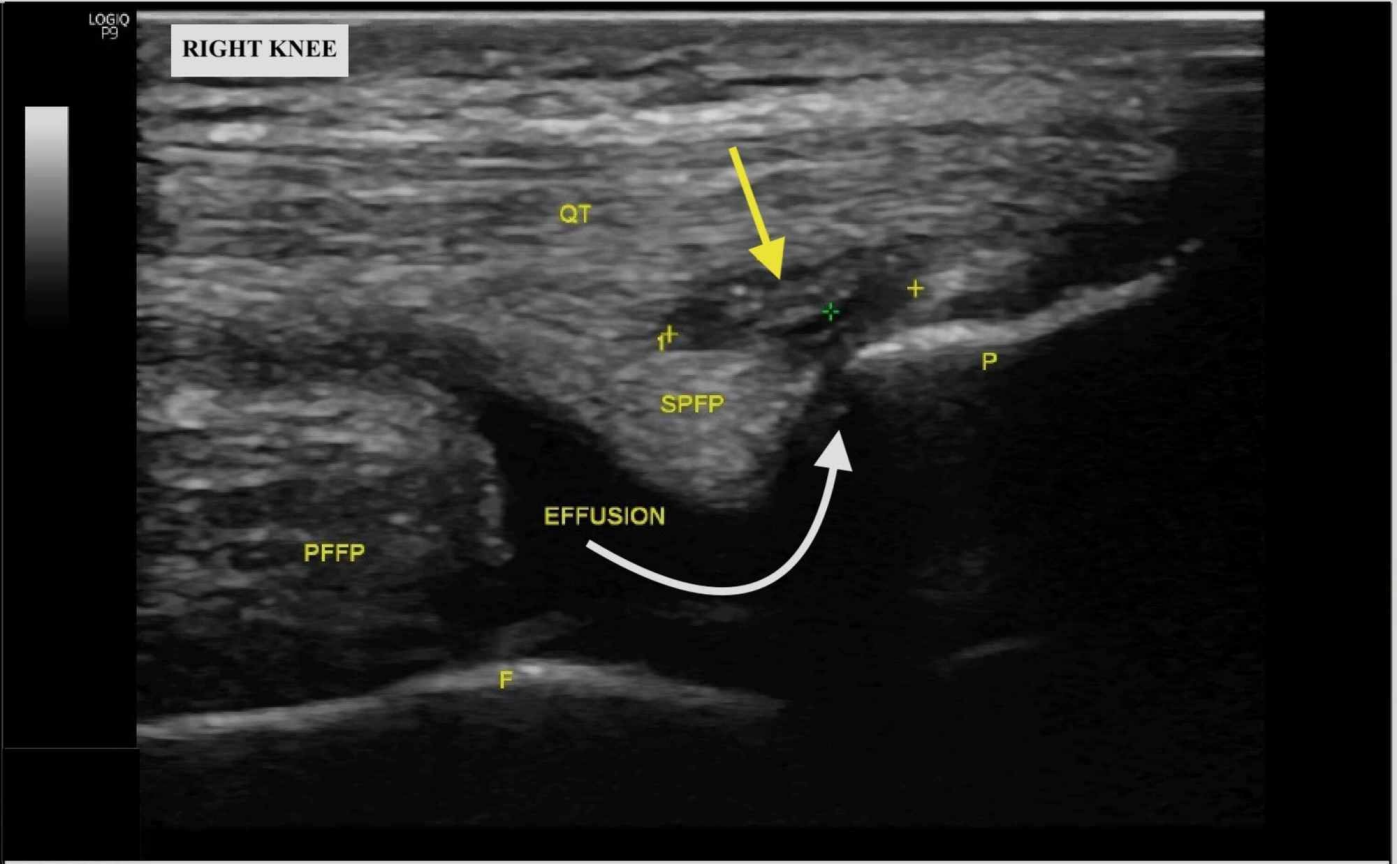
Long-axis ultrasound image of the right knee at three-month follow-up; white arrow demonstrates the path of a suprapatellar effusion communicating into the vastus intermedius fibers of the right quadriceps tendon (yellow arrow) QT: quadriceps tendon; PFFP: prefemoral fat pad; SPFP: suprapatellar fat pad; F: femur; P: patella
